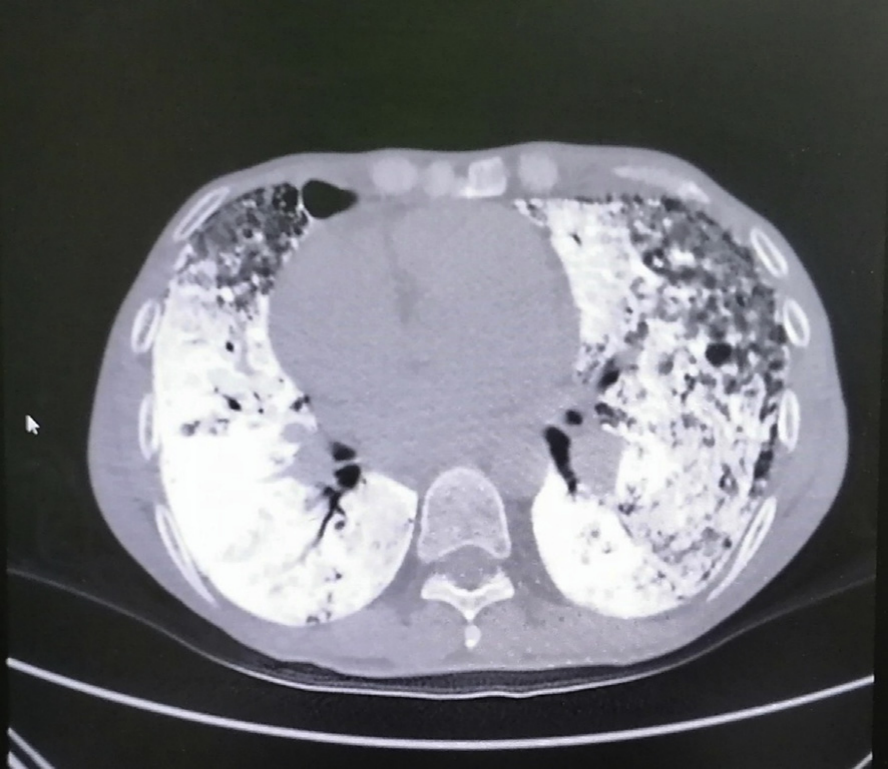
High-resolution CT scan of the chest showing the classical crazy-paving appearance of pulmonary alveolar microlithiasis.
